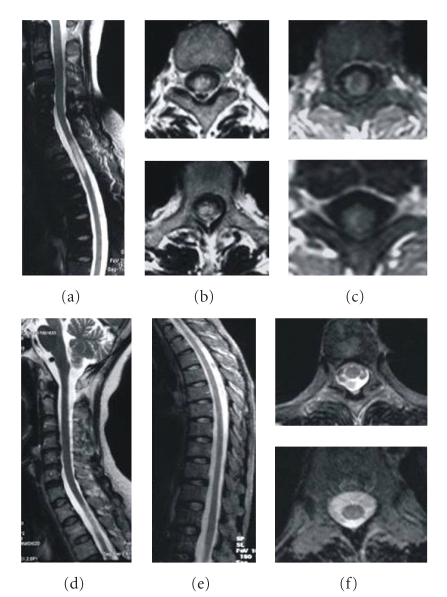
A 58-year-old female developed paraplegia and paresthesias in August 2007; weakness slowly worsened. Pulse methylprednisolone and IVIG were initiated with no resolution; she continued to deteriorate. After 2-year ATT, her situation fully recovered. The MRI changes are as follows: (a) sagittal T2-weighted image showing a contiguous area of increased signal intensity spanning T2 to T3 level, (b) axial T2-weighted images reveal the strong signals, (c) axial MRI images showing focal cord enhancement, (d) after 5 months of ATT, the lesion decreased, (e) following 1-year ATT, sagittal T2-weighted MRI of the thoracic cord reveals no abnormal signals, and (f) no lesion is visible on cross-sectional T2-weighted images.
